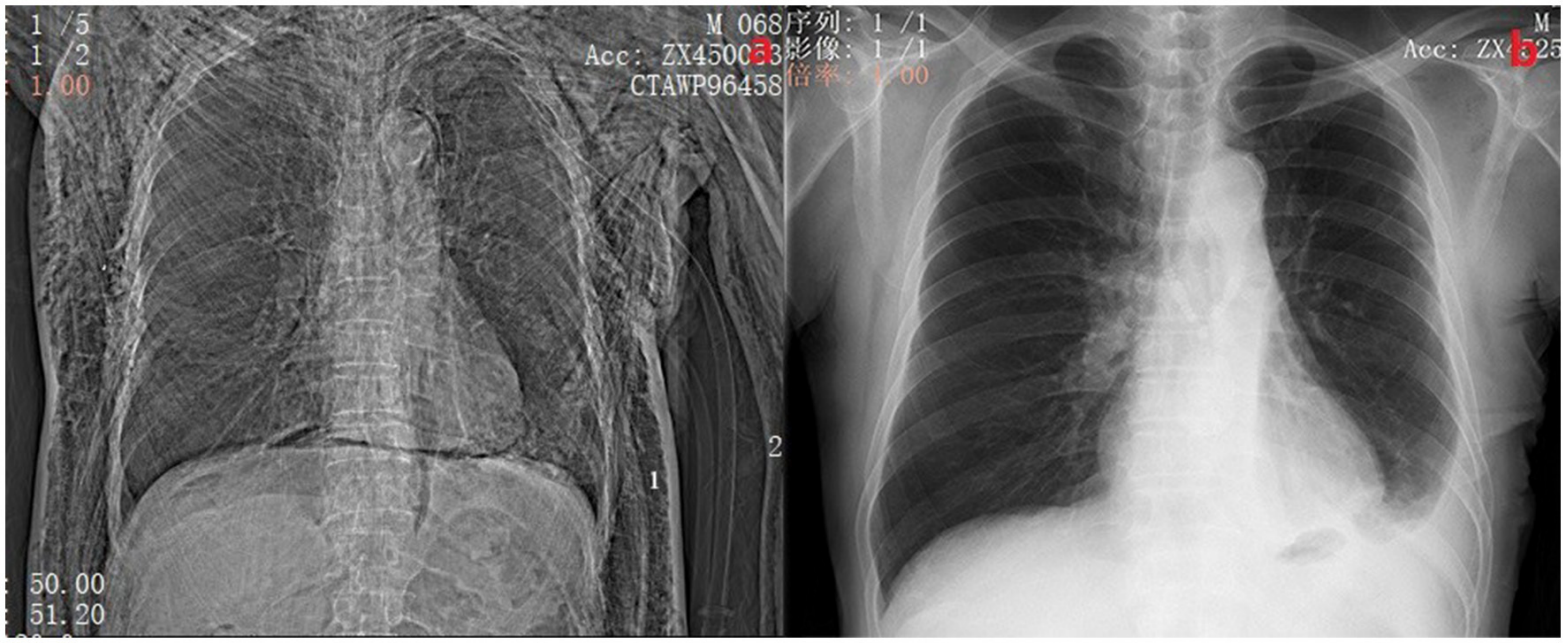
(A) Shows a patient with postoperative pneumothorax and subcutaneous emphysema with 2 chest tubes inserted. The side marked with 1 is the chest tube placed during surgery, and the side marked with 2 is the chest tube placed at the bedside. (B) Shows a chest X-ray of the same patient 2 weeks after discharge.
